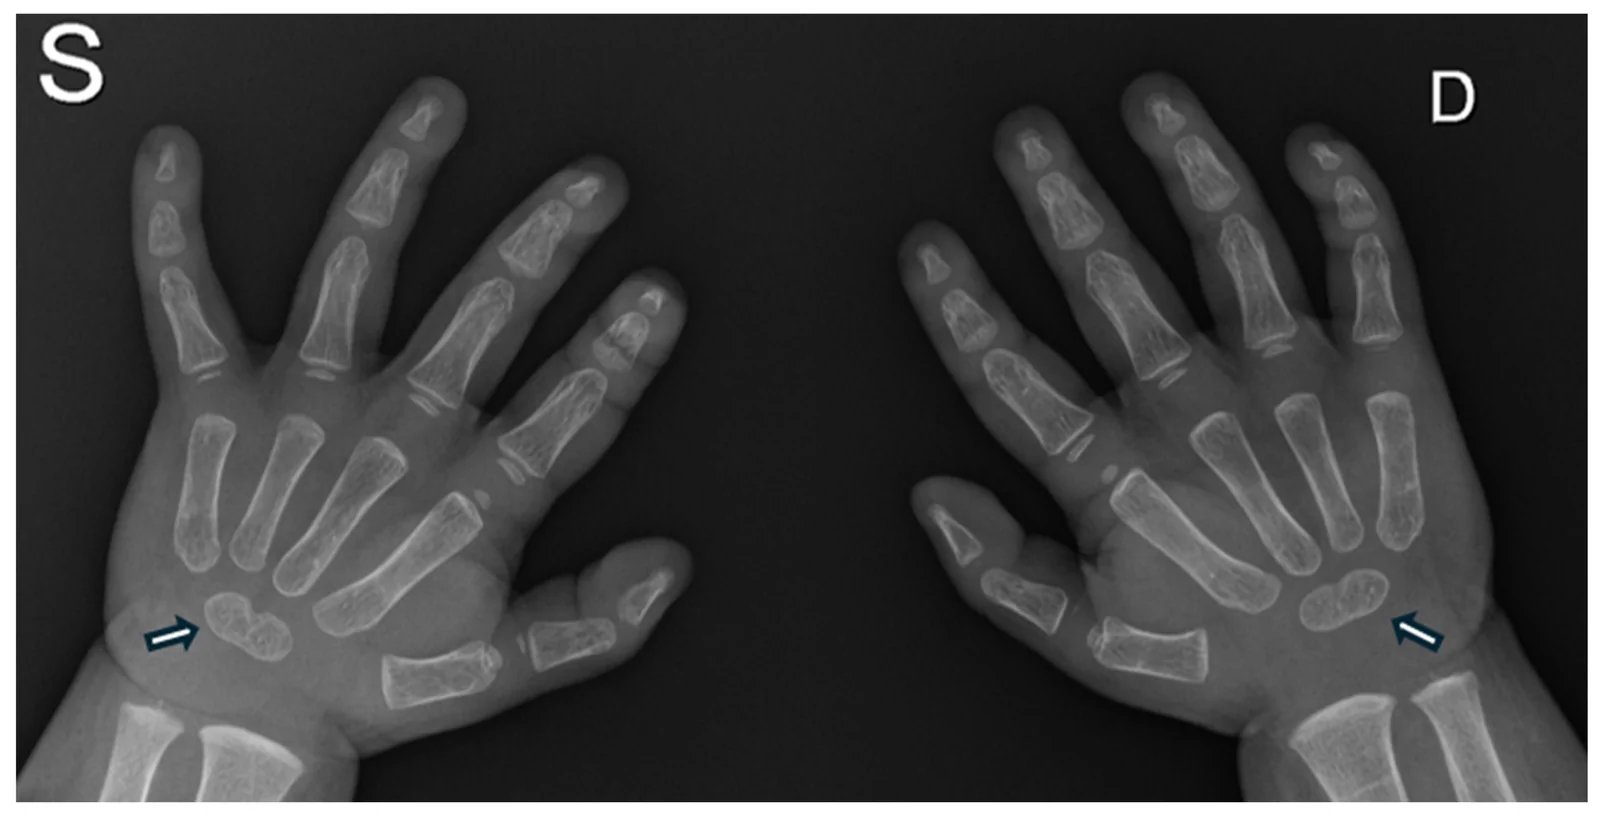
Hand radiograph at 2 years and 4 months of age demonstrating delayed bone age (assessed as approximately 9 months by the Greulich and Pyle method) and fusion of the capitate and hamate ossification centers (arrow), consistent with the carpal synostosis characteristic of *FLNB*-related SCT.
